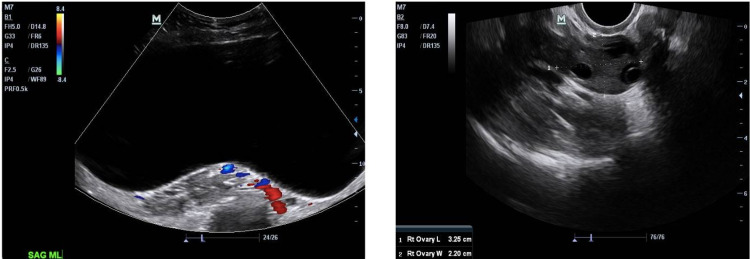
Preoperative (left-benign serous cystadenoma) and postoperative ultrasound (right-normal) images of the patient.
